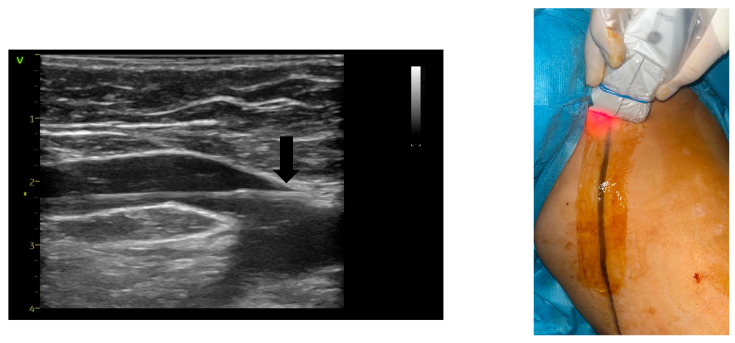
Catheter tip (arrow) positioning at the saphenofemoral junction using ultrasound guidance (left) and pilot setting guidance (right).
